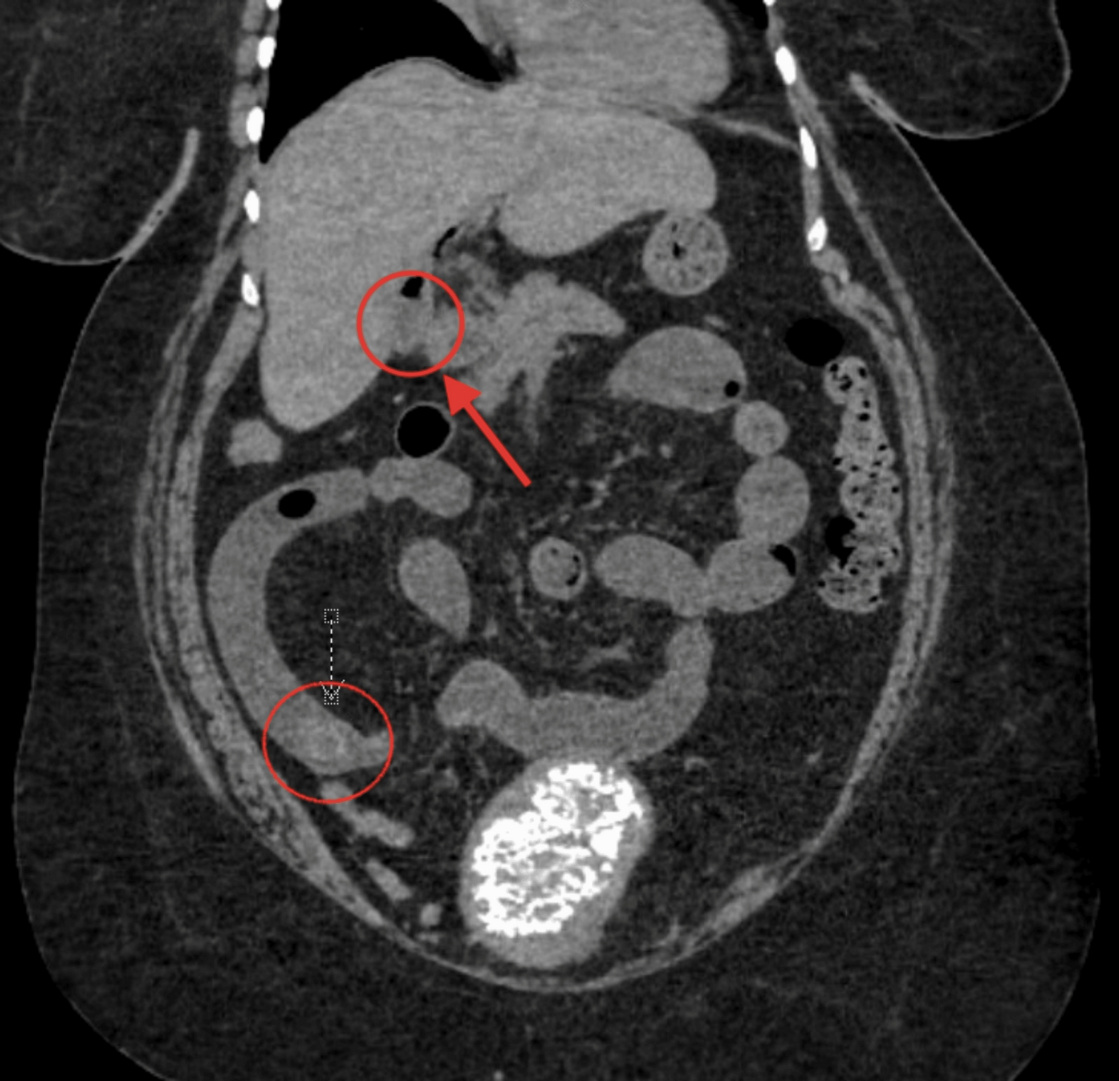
CT demonstrating an intraluminal gallstone in the distal ileum (circled in red) and a cholecystoenteric fistula (red arrow) with no remaining stones in the gallbladder
